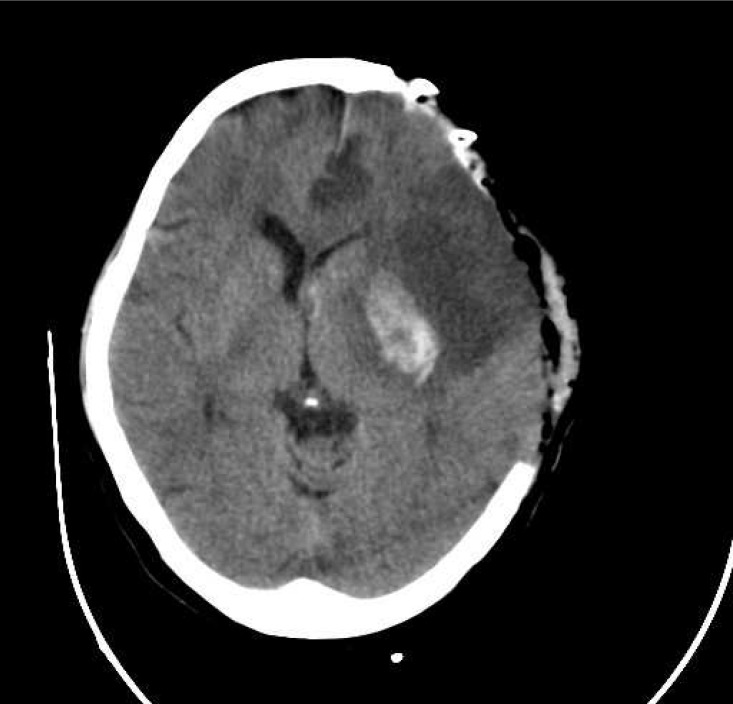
CT scan after decompressive craniectomy
